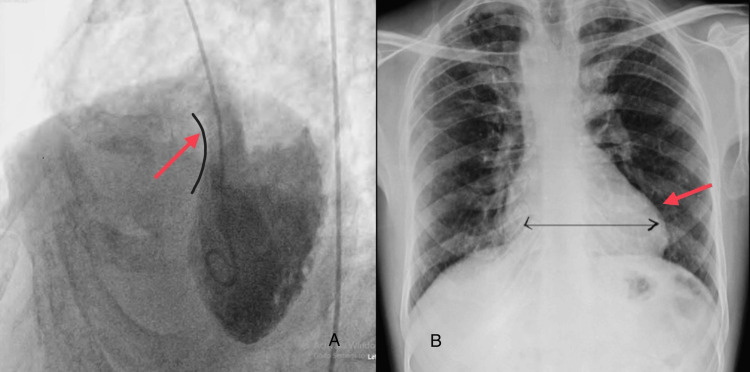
(A) The angiogram shows a malaligned sub-aortic ventricular septal defect (indicated by the red arrow pointing toward the black curve of the ventricle); (B) the postero-anterior view of the chest X-ray shows a boot-shaped heart with dilated ventricles (as marked by the red arrow) The images were taken by the authors
